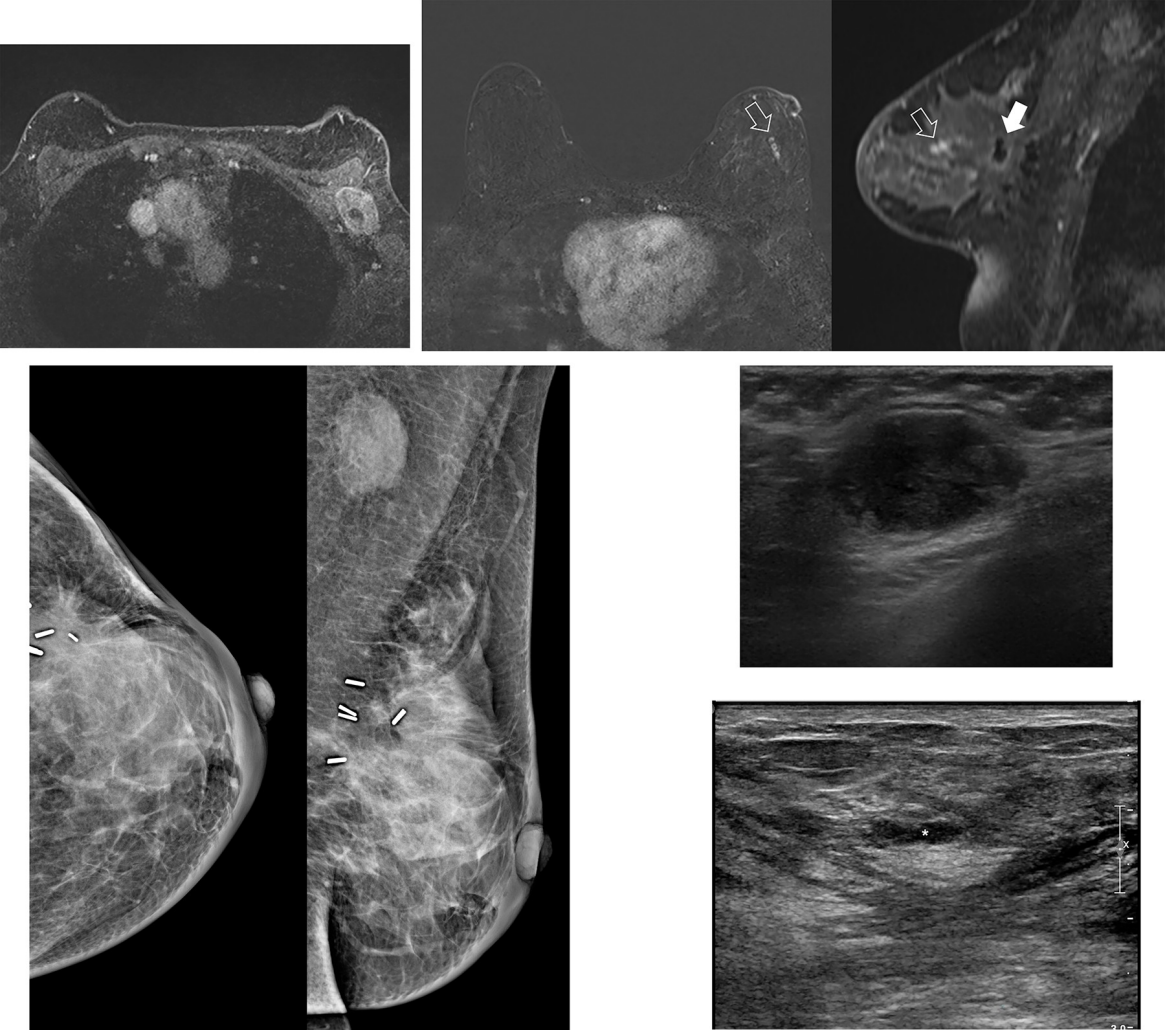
A 40-year-old female patient with an early recurrent breast cancer detected 8 months after BCT. The patient who underwent BCT for left breast cancer (invasive breast cancer, stage II) complained of discomfort in left axilla at the time of postoperative surveillance. Through mammography and ultrasound, an enlarged lymph node was noted. It was confirmed as metastasis by ultrasound-guided core needle biopsy. (a) MRI after confirmed metastatic lymph node in left axilla. A large lymph node, which was confirmed as metastasis, is noted at left axillary level I. (b) At first dynamic phase after contrast enhancement, there is a focal non-mass enhancement (open arrow) at 3 o’clock direction of left breast, anterior to the previous excision site (white arrow). (c), (d) Mammography and ultrasound of the same patient show an enlarged lymph node in left axilla, but there is no significant suspicious finding at excision site on both mammography and ultrasound. (e) On second look ultrasound by breast expert to find non-mass enhancement lesion detected on MRI, an indistinct isoechoic non-mass like lesion (white star) is noted. This lesion was confirmed to be recurrent invasive carcinoma after re-excision with ultrasound-guided needle localization.
